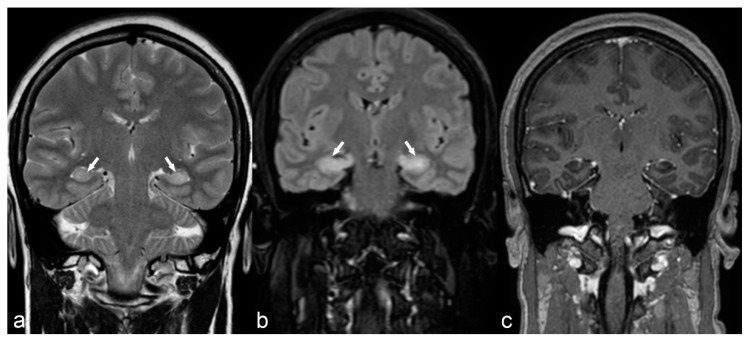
50-year-old woman. Symmetrical hyperintensity is identified on coronal T2WI (a) and FLAIR (b) in both hippocampi (arrows). No abnormal enhancement is seen on 3D T1 Gd (c). This is a case of autoimmune limbic encephalitis.
